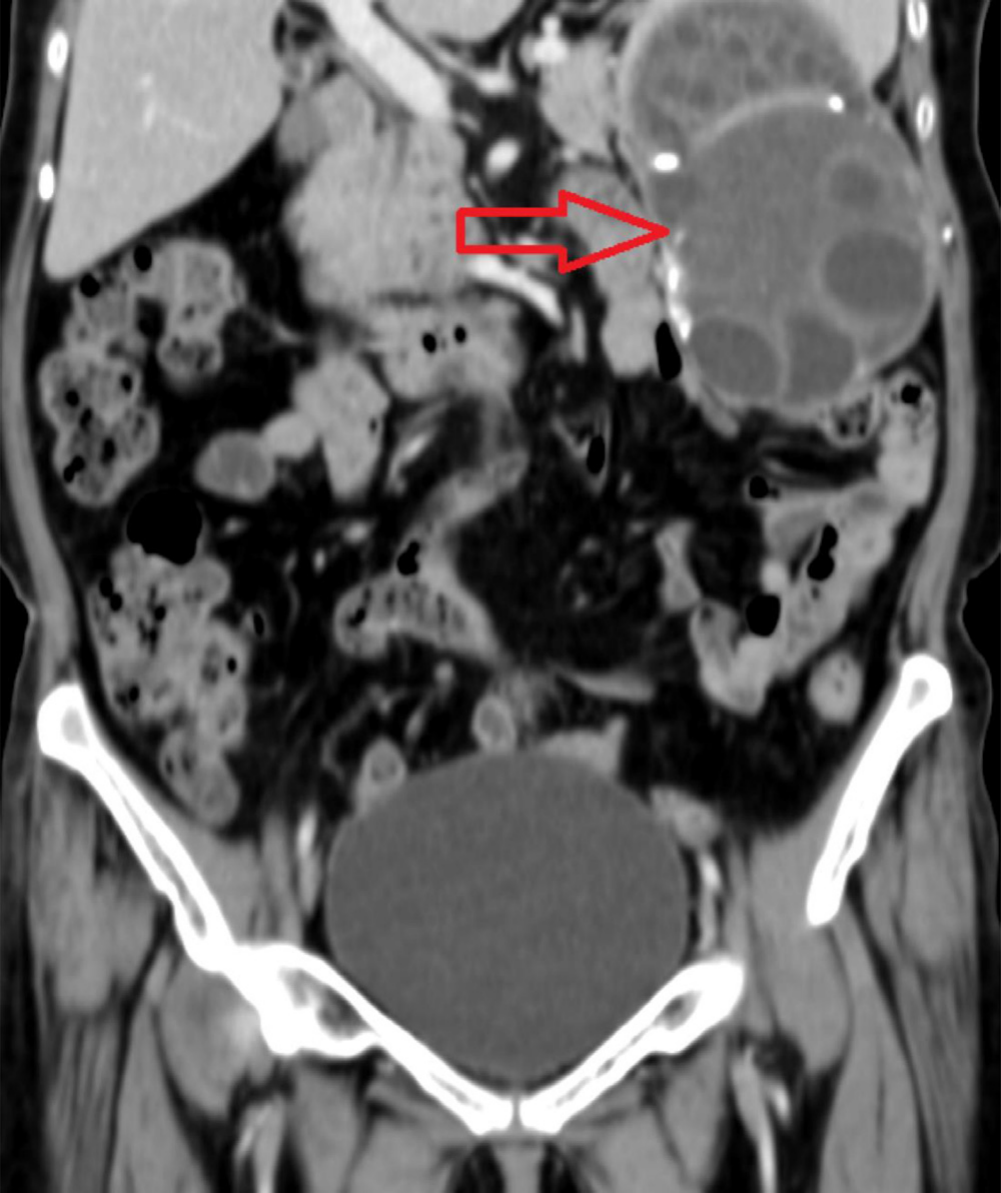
CT scan coronal window showing multiloculated hypodense cyst multiple peripherally located daughter cysts. Wall of the lesion was partially calcified. The lesion measured 14 × 7.7 × 13.2cm.
